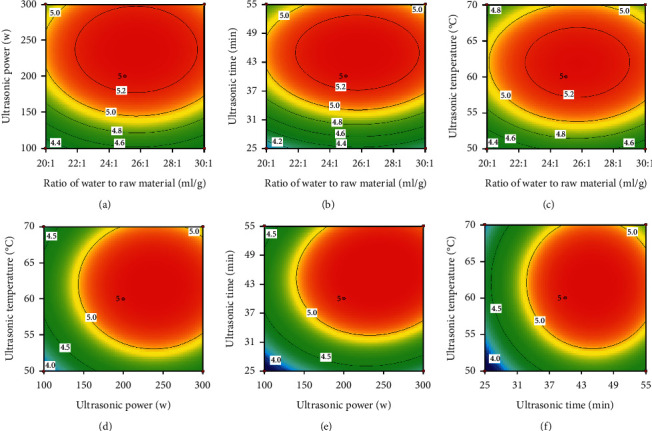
Contour plots showing the interactive effects from the ratio of water to raw material, ultrasonic power, ultrasonic time, and ultrasonic temperature on extraction yield of PBOM.
